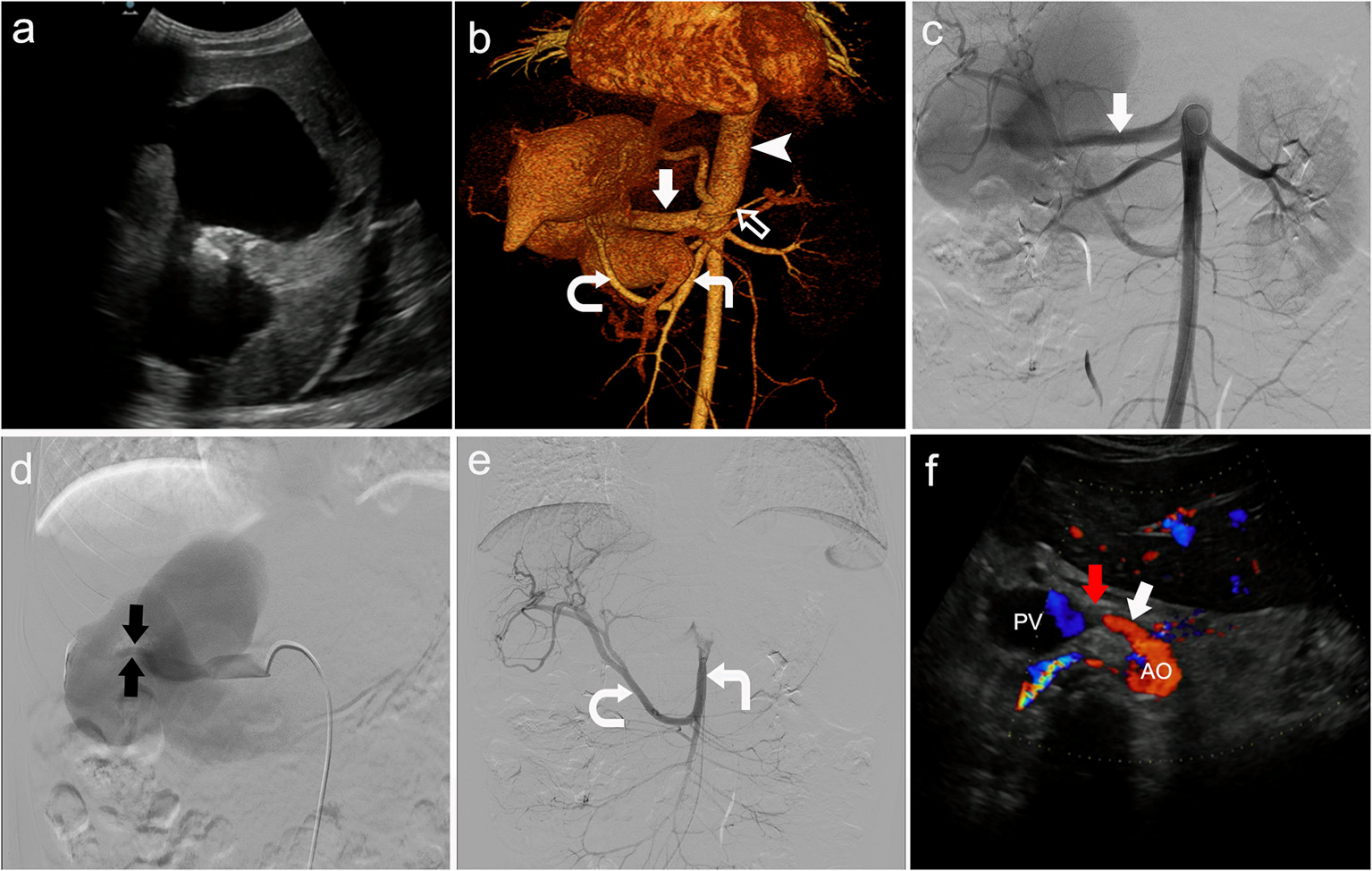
Congenital visceral vascular variation was diagnosed by multi-imaging examinations. (a) Tumor-like expansion of the main portal vein and the left branch of the portal vein shown by color Doppler ultrasound. (b) Contrast-enhanced computed tomography scan showed the left hepatic artery (black arrow) and left gastric artery (not shown) co-trunk, as the first branch of the aorta (arrowhead). The common hepatic artery (straight solid arrow) and splenic artery (hollow arrow) originated from the front wall of the aorta below the left hepatic-gastric trunk, while the right hepatic artery (curved arrow) originated from the superior mesenteric artery (right angle arrow). (c) Angiogram showed the common hepatic artery (straight solid arrow). (d) Angiogram showed the fistula (the tiny blood vessels between the two black arrows). (e) Angiogram showed that the right hepatic artery (curved arrow) originated from the superior mesenteric artery (right angle arrow). (f) The position of the occluder (red arrow) was normal and the fistula was completely closed, 3 months after surgery.
